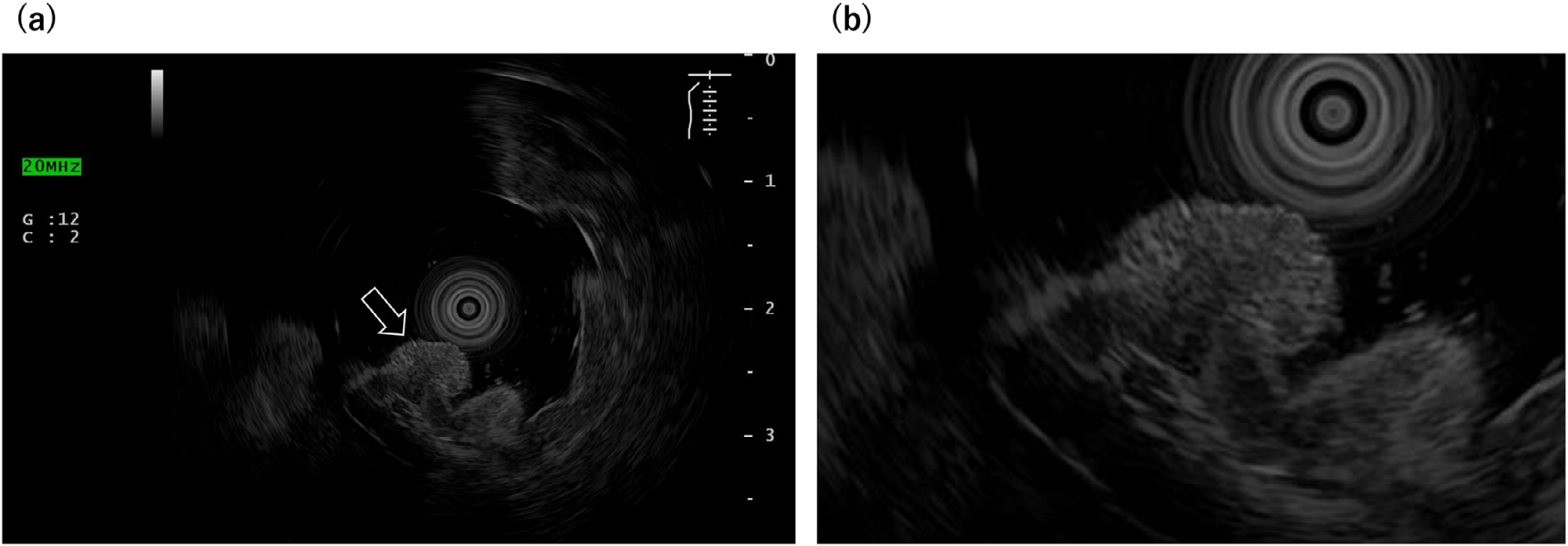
Endoscopic ultrasonography (EUS). (a) A hypoechoic, 4‐mm lesion localized to the second layer of the gastric wall. (b) The third layer remained intact, with no thinning or disruption, indicating the confinement of the lesion.
